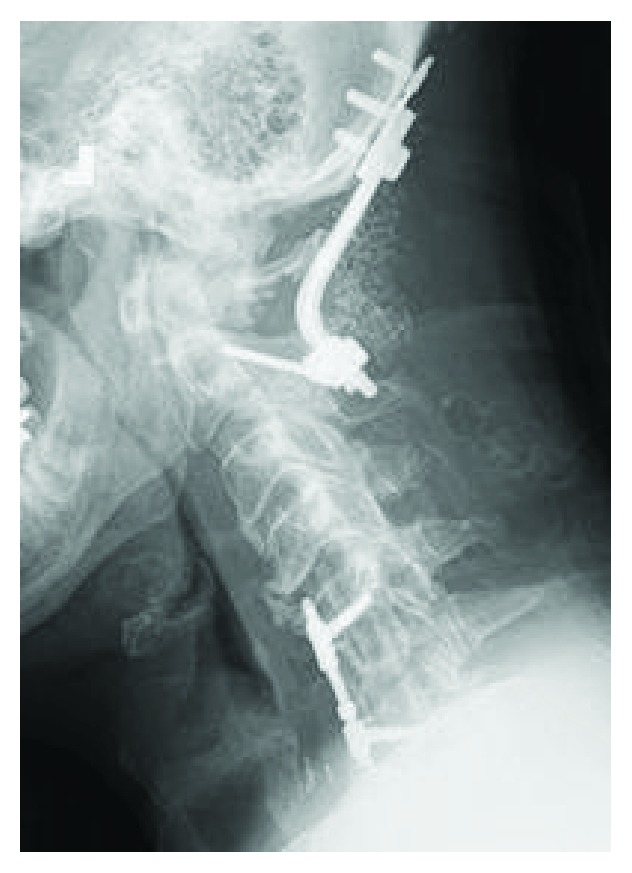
Postoperative lateral radiograph: occiput-C2 fixation with bilateral C2 pars screws and occiput plate connected with precontoured occiput cervical rods. Past surgical history of C5–C7 anterior arthrodesis.
